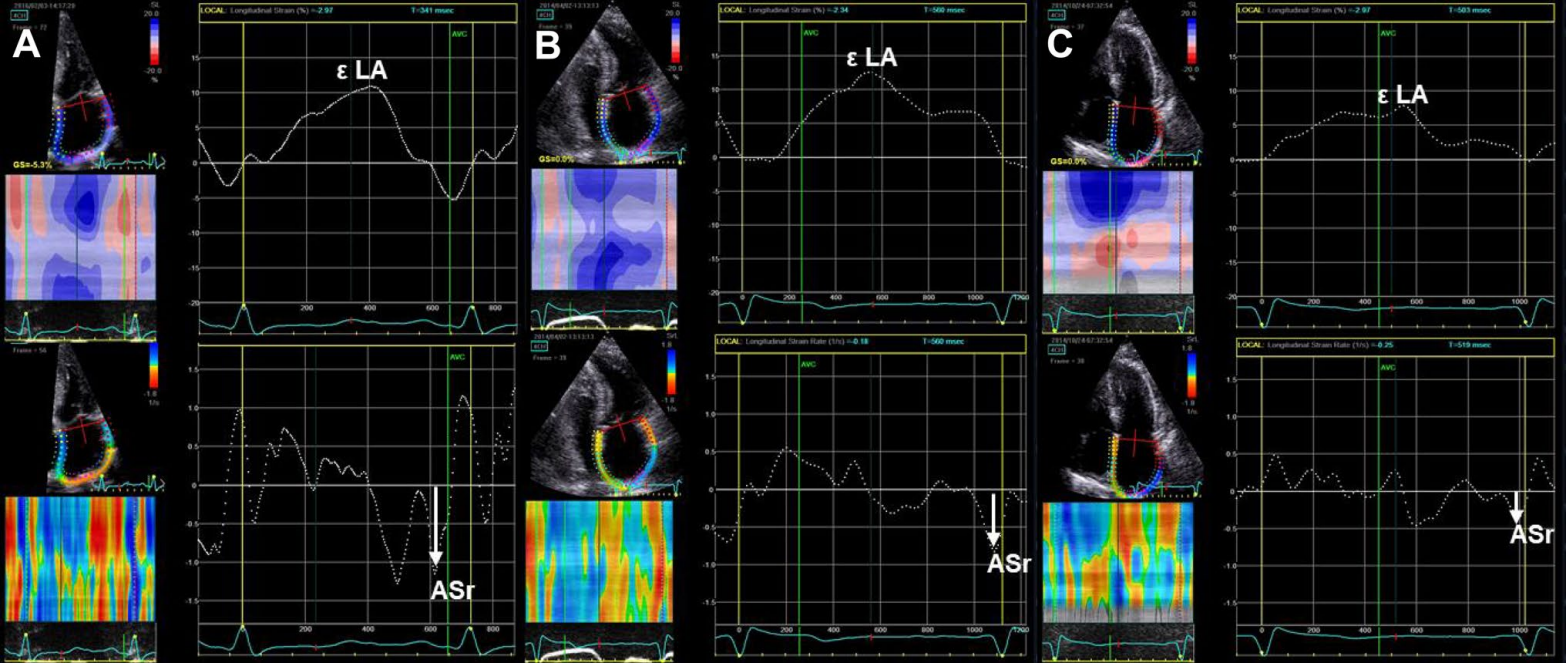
The assessment of LA function (reservoir function—ƐLA, and booster pump function—ASr) by speckle tracking echocardiography in three patients with HCM: a A patient with HCM, with anterior–posterior LA diameter < 45 mm, and without history of AF. b A patient with HCM, with anterior–posterior LA diameter < 45 mm, and with paroxysmal AF. c A patient with HCM, with anterior–posterior LA diameter > 45 mm, and with paroxysmal AF. Note that LA booster pump function is reduced in HCM patients in the presence of paroxysmal AF, furthermore if there is significant LA dilation (> 45 mm). *ƐLA* global longitudinal LA strain, *ASr* peak late diastolic strain rate, *LA* left atrium, *AF* atrial fibrillation, *HCM* hypertrophic cardiomyopathy, *DAS* anteroposterior LA diameter
